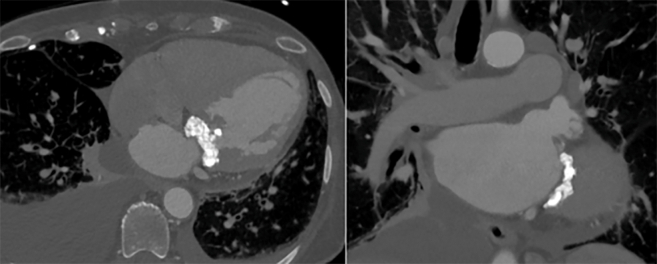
Computed tomography of the chest demonstrating extensive mitral annular calcification.
